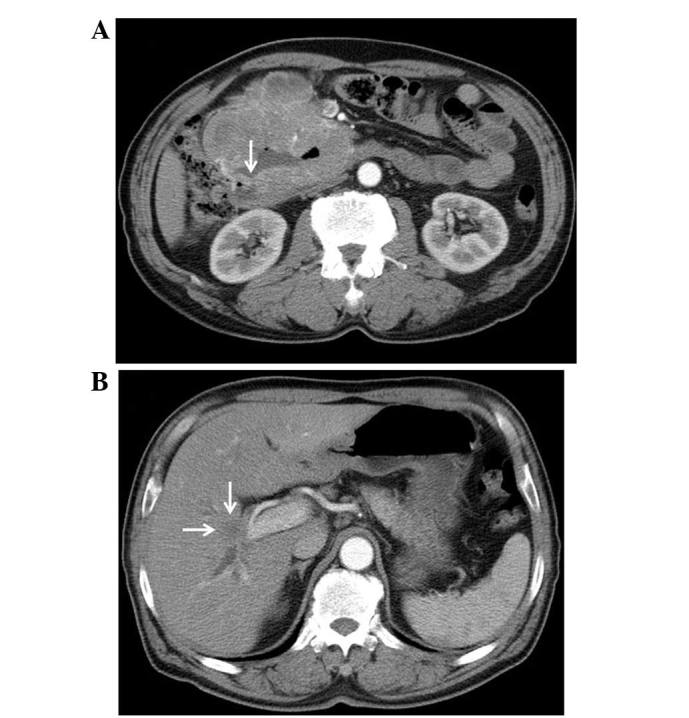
Computed tomography scan of the abdomen upon admission to hospital. (A) Large lobulated and heterogeneously enhanced mass (11.5×9.3 cm) with internal necrosis. An air-fluid level was noted on the pancreaticoduodenal groove and the internal cavity of the mass was connected to the second portion of the duodenum (arrow). (B) An Ill-defined low density lesion was noted at hepatic segment eight, abutting the bile duct and hepatic artery. The right intrahepatic duct was marginally dilated by the lesion.
